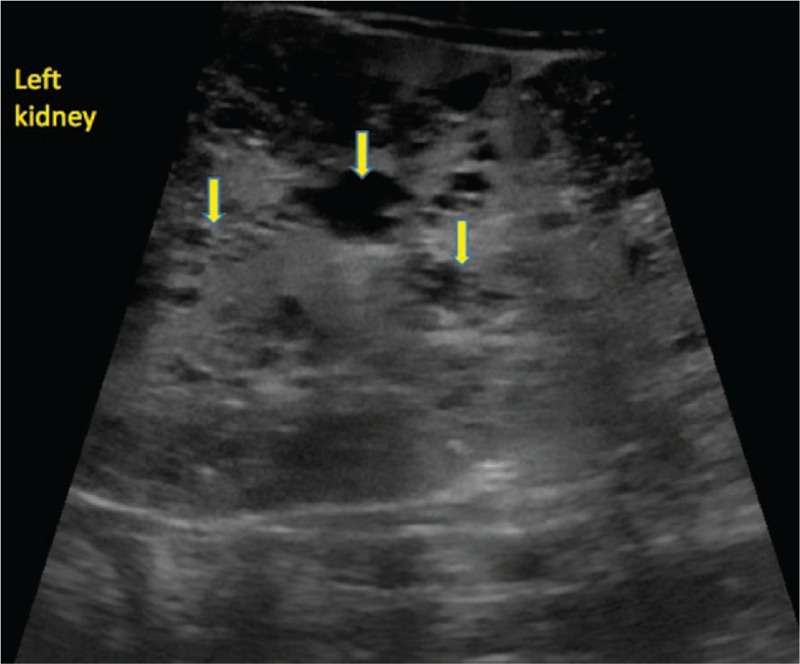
The evolution of the cystic lesions in the left kidney (Ultrasound aspect - 1 year and 2 months of age).
